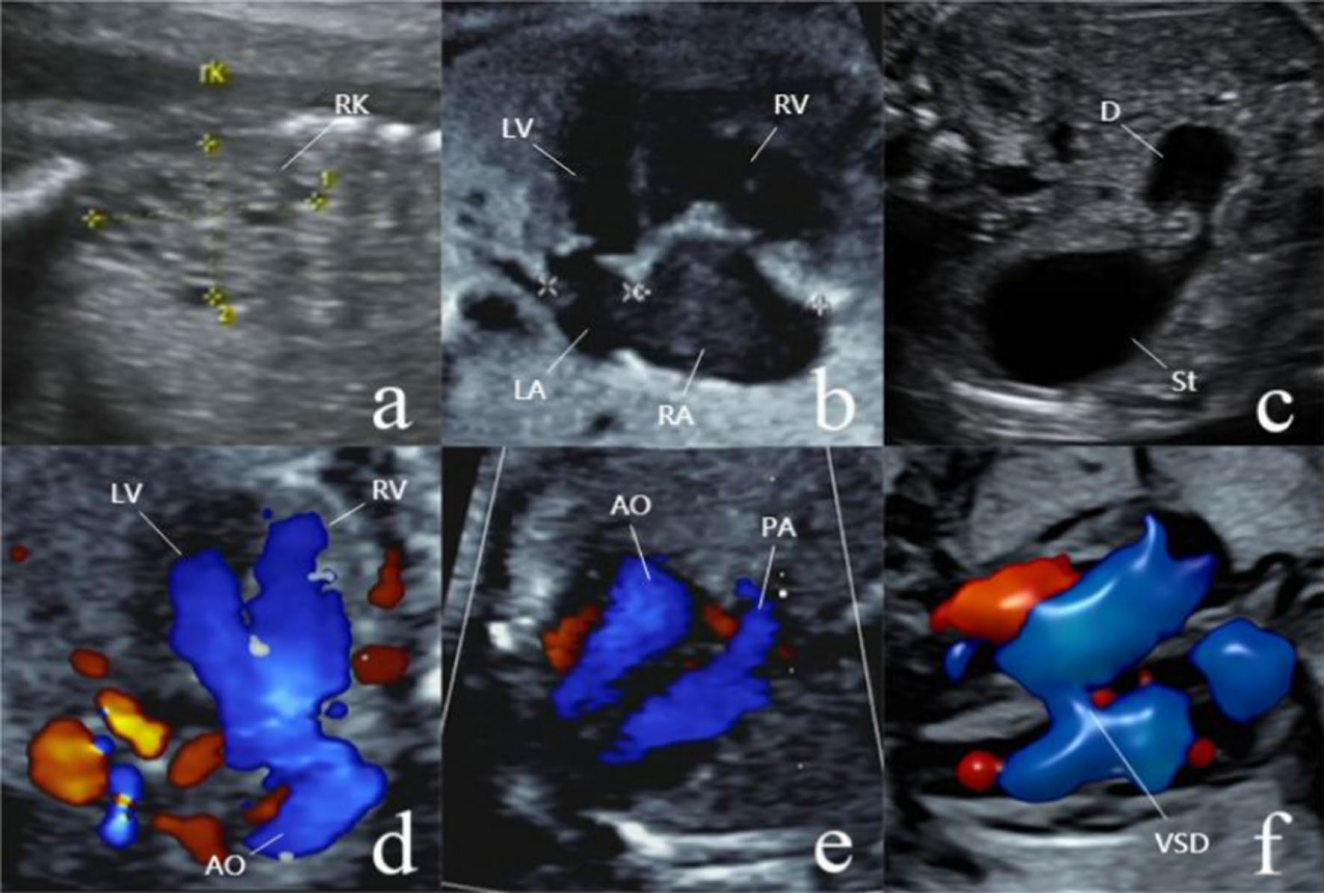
Some typical ultrasound performance in these cases. a The right kidney (RK) of Case 1 showed multicystic dysplastic kidney. b Case 2 showed atrioventricular ratio is out of balance. c The ultrasound image of Case 3 showed the characteristic double bubble sign. d The image of Case 4 showed that two blue blood flow signals of the left and right ventricles respectively enter the aorta. e Case 5 showed mild stenosis of the pulmonary artery. f On the Four-Chamber View, Case 8 showed that the blood flows across the septum. *LV* left ventricle, *LA* left atrium, *RV* right ventricle, *RA* right atrium, *D* duodenum, *St* stomach, *AO* aorta, *PA* pulmonary artery, *VSD* ventricular septal defect
